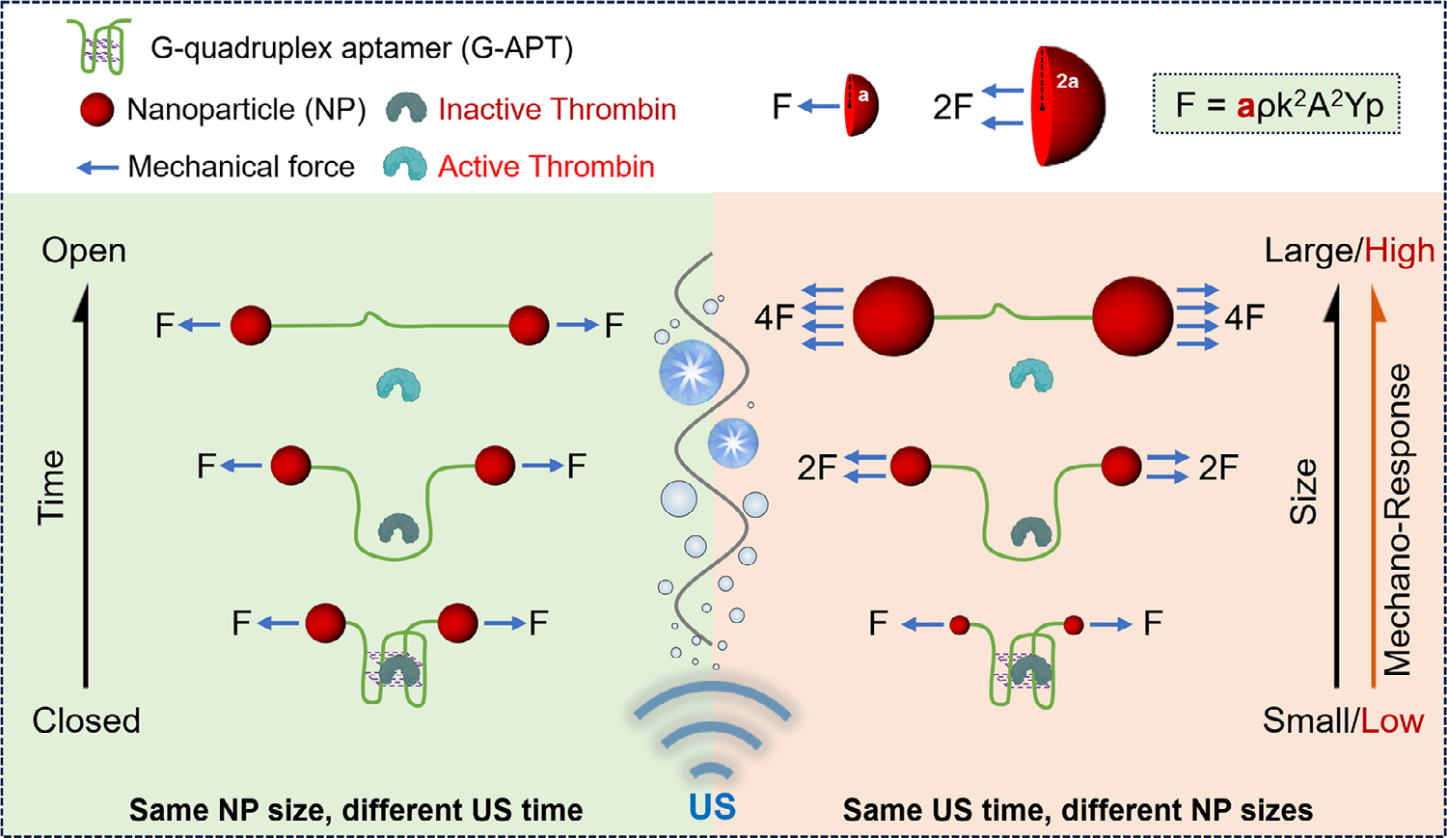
Size‐dependent ultrasound activation of the thrombin catalytic activity by mechano‐nanoswitches.
